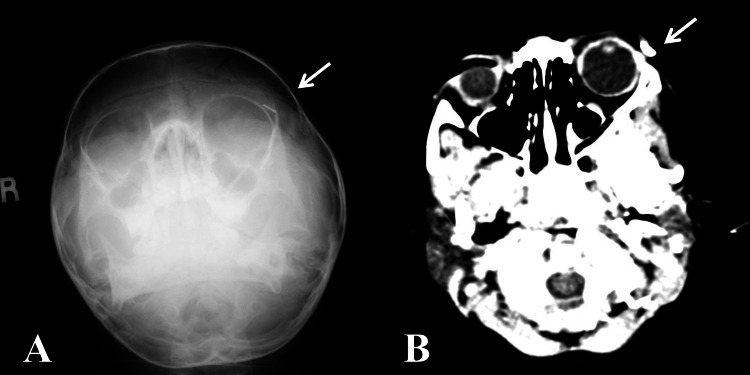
The foreign body (white arrow) in (A) Water's view skull x-ray and (B) CT
